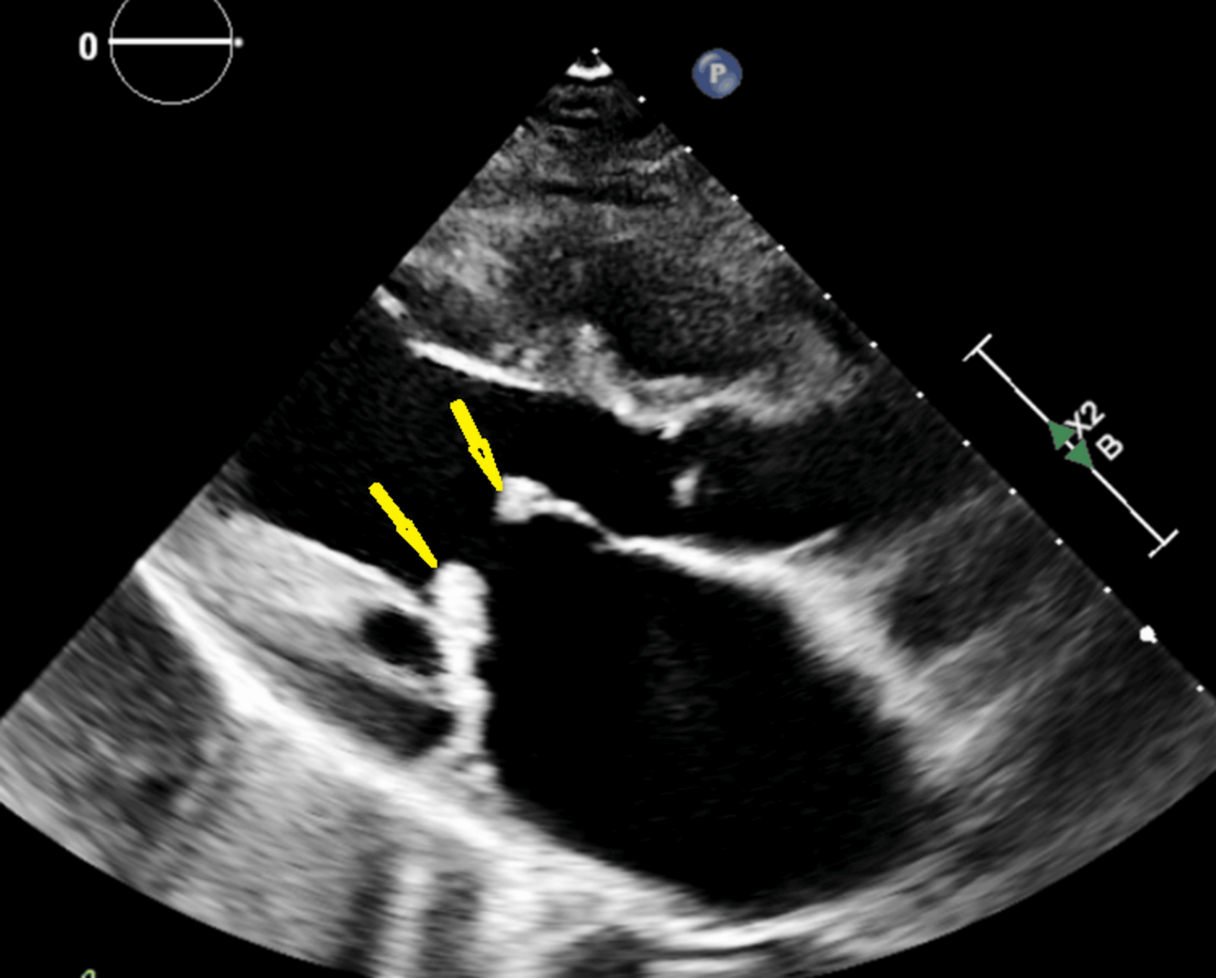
Transthoracic echocardiography at presentation Transthoracic echocardiography demonstrating thickened mitral valve leaflets with irregular echogenic masses on the atrial surfaces (yellow arrows), associated with severe mitral regurgitation, consistent with Libman-Sacks endocarditis at presentation.
